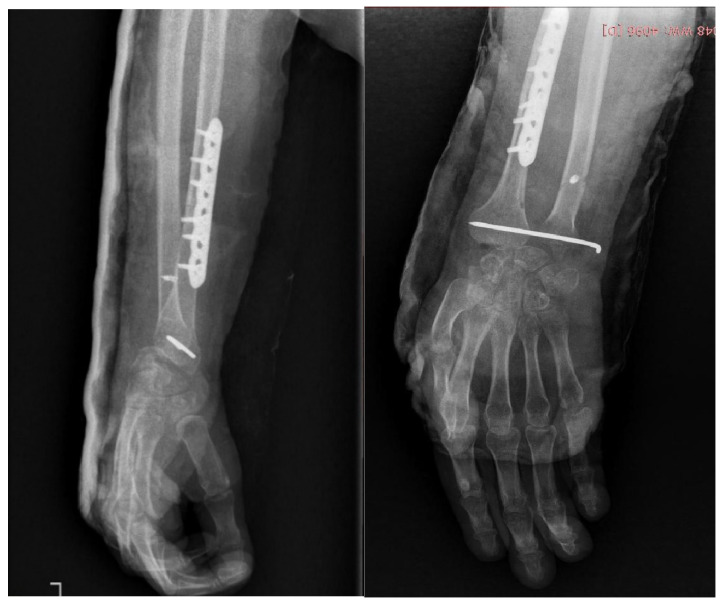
Postoperative control radiographs (anteroposterior and lateral views) of the wrist joint. The lateral view demonstrates early postoperative dorsal subluxation of the carpal bones, while the anteroposterior view shows correct positioning of the proximal fibular graft and plate osteosynthesis with K wire in its correct place.
